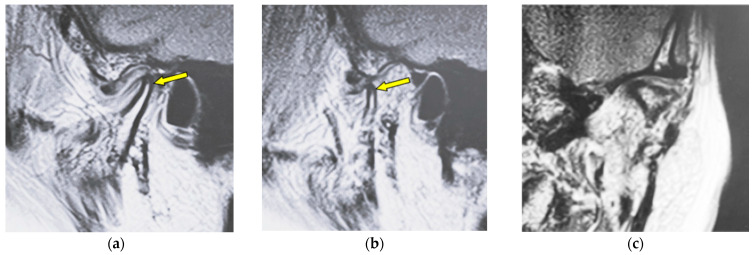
MR image (proton-density-weighted) of an 82-year-old woman (disc displacement without reduction, left disc): (a) sagittal oblique cross-section imaging in the mouth-closing position; (b) sagittal oblique cross-section imaging in the mouth-opening position; (c) coronal oblique cross-section imaging in the mouth-closing position. The arrow shows the part with atrophy.
